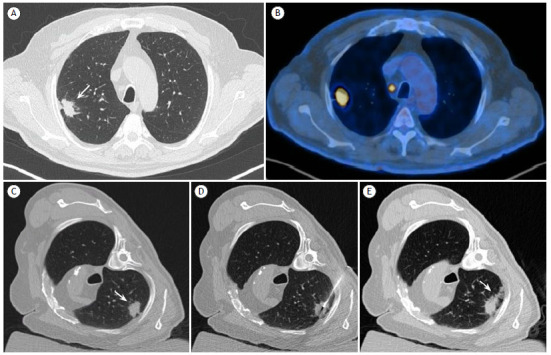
(A) Non-contrast chest computed tomography (CT) showing a non-calcified pulmonary nodule with spiculated margins (white arrow) located in the peripheral region of the posterior segment of the right upper lobe. The lesion measures approximately 2.3 × 1.2 cm in its largest axial dimensions and is adjacent to the parietal pleura, suggestive of primary lung neoplasia. (B) PET-CT with 18F-FDG showing intense glycolytic activity in the pulmonary nodule (SUVmax: 12.5), in addition to marked radiotracer uptake in a mediastinal lymph node in the right paratracheal chain (SUVmax: 10.8), suggestive of secondary involvement. (C) Chest CT during pre-procedural planning, with the patient in the right oblique lateral decubitus position. (D) Intra-procedural chest CT showing placement of the 19-gauge coaxial cannula via posterior access. (E) Post-procedural control chest CT showing the blood patch along the path of the coaxial cannula (white arrow).
